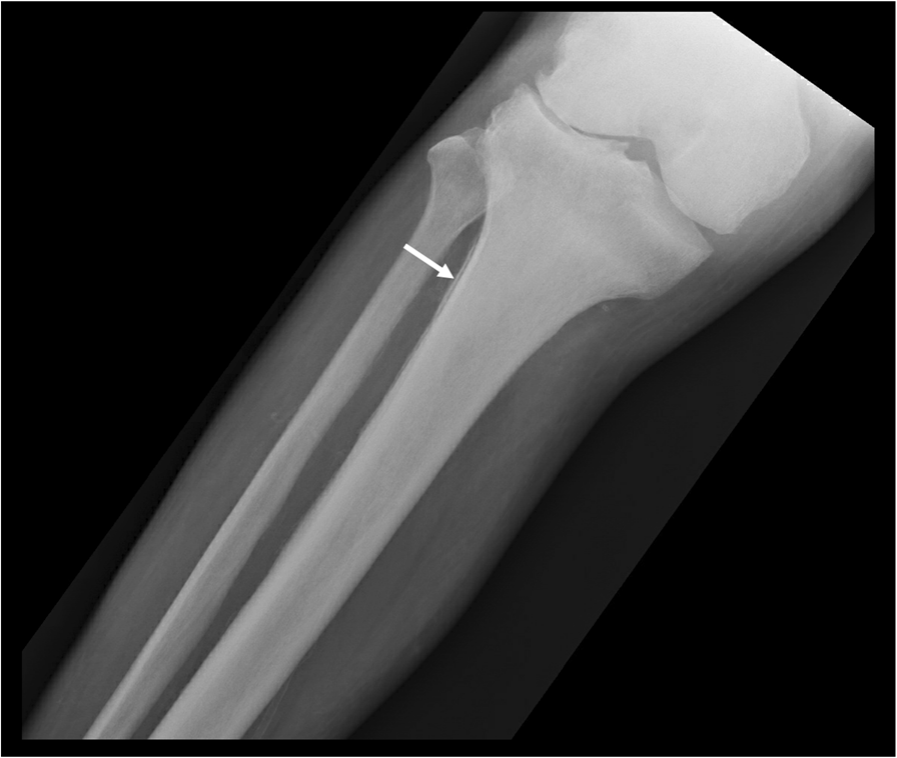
AP radiograph in the same patient demonstrates mild periostitis at the proximal tibial metaphysis, a rare feature of myelofibrosis (white arrows). Periostitis in myelofibrosis occurs at the metaphyseal regions of the distal femur or proximal tibia
